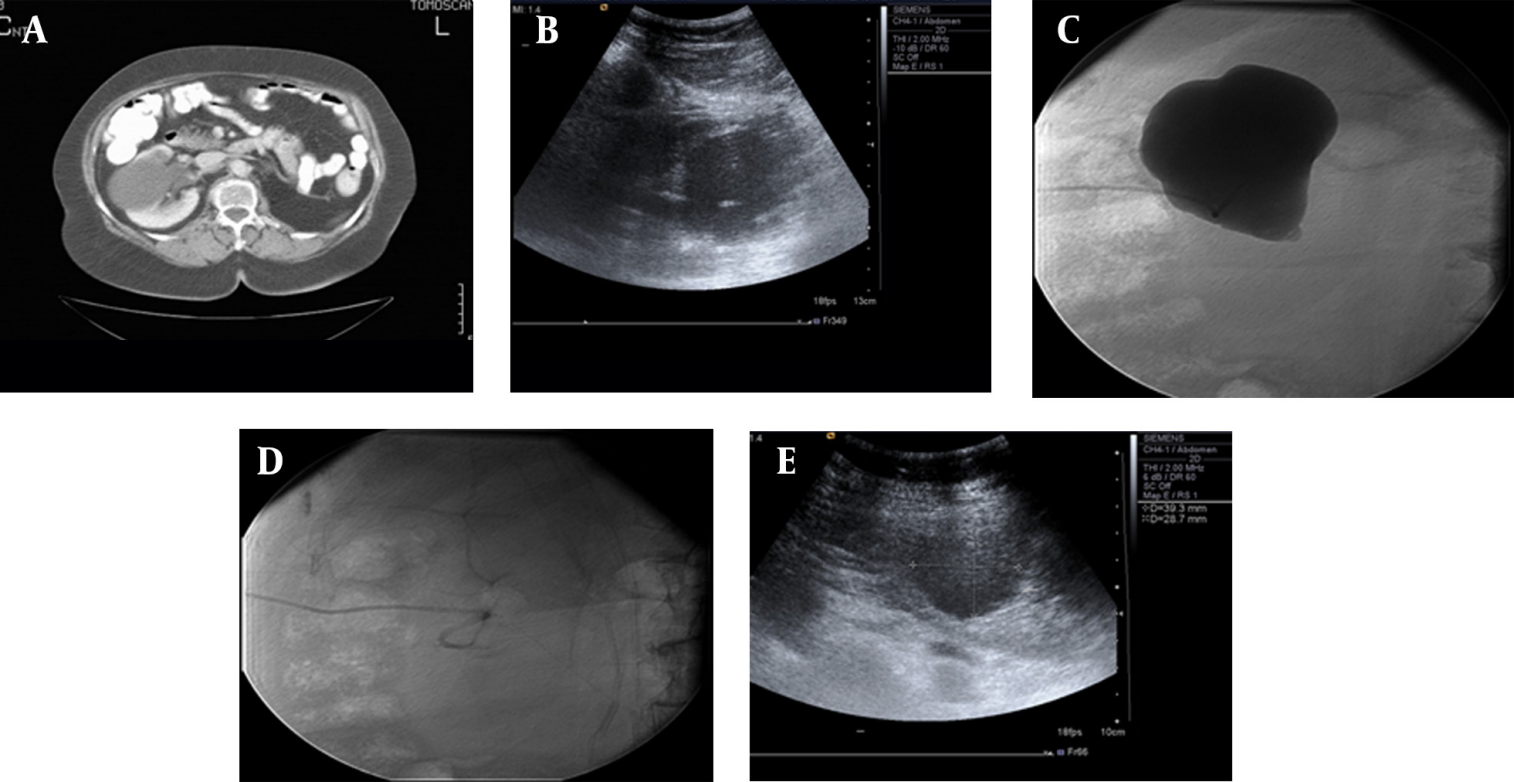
A 56-year-old man with Bosniak type-1 cyst in the right kidney with flank pain. A) Axial CT image shows Bosniak type-1 cyst in the right kidney which has affected the pelvicalyceal system; B) US image shows the initial catheterization of the choice-lock catheter in the cyst during the treatment procedure; C) Cystogram shows that the cyst does not have any relationship with the pelvicalyceal system; D) Cystogram obtained after emptying of the cystic fluid; E) US image shows partial regression of the cyst one month after treatment.
